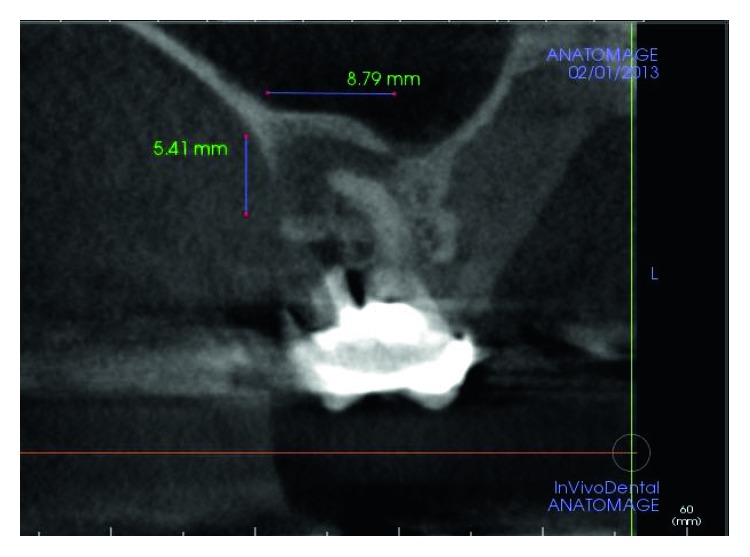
A cone beam CT of tooth #3 in 2013 showed periapical radiolucency of the mesial buccal root (∼5.4 × 8.8 mm).
